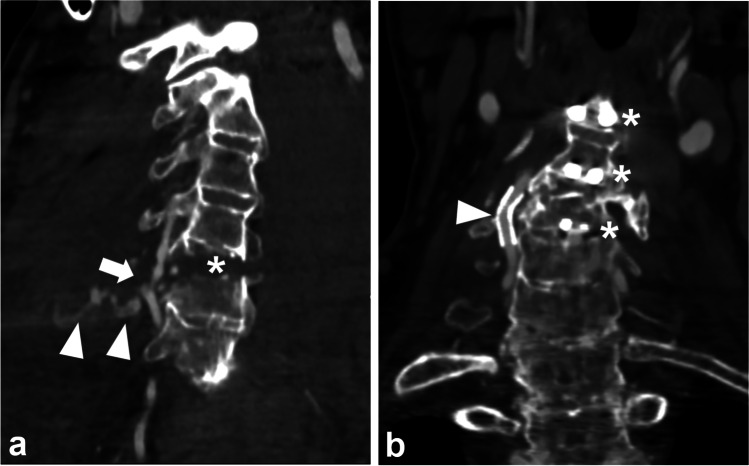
After falling from a bicycle, a patient aged 57 was found by his neighbor, developed swelling around the neck, and had difficulties in breathing caused by active extravasation (arrowheads) from a grade V BCVI in the right vertebral artery (arrow) shown in CTA of 2 mm thickness coronal slices. The unstable cervical spine fracture (asterisk) disrupted the intervertebral disc between the fourth and fifth cervical vertebrae in the ankylosed spine caused by ankylosing spondylitis (a). The BCVI was treated with an endovascular stent. On follow-up CTA, the stented vertebral artery (arrowhead) remained open without any ischemic lesions. Anterior fixation screws (asterisks) are shown in the third, fourth, and fifth vertebrae (b). BCVI, blunt cerebrovascular injury; CTA, CT angiography
